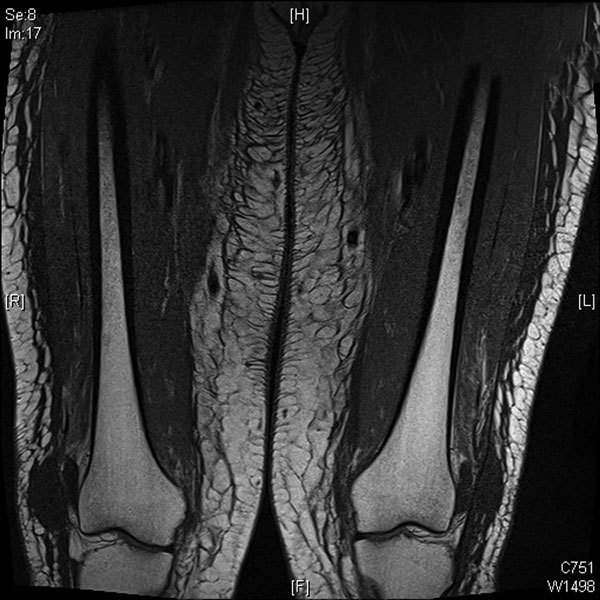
Magnetic resonance image of 63-year-old woman in Australia with disseminated enteroviral infection that manifested after she received obinutuzumab for lymphoma. Image shows patient’s thighs and diffuse inflammatory changes involving subcutaneous tissues, fascia, and musculature.
